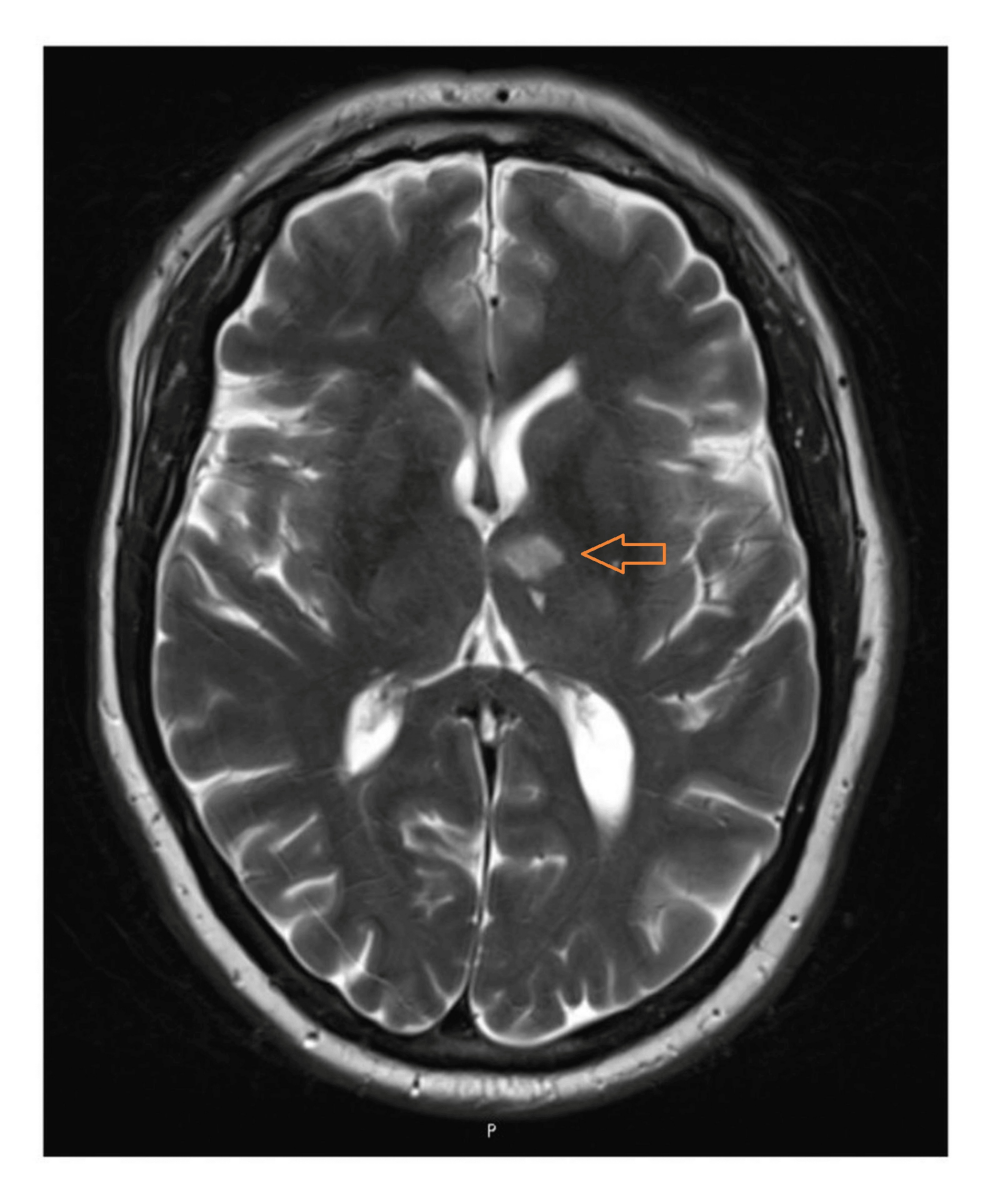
Magnetic Resonance Imaging (MRI) of the head Clinical photograph at presentation showing an anterior thalamic infarct.
